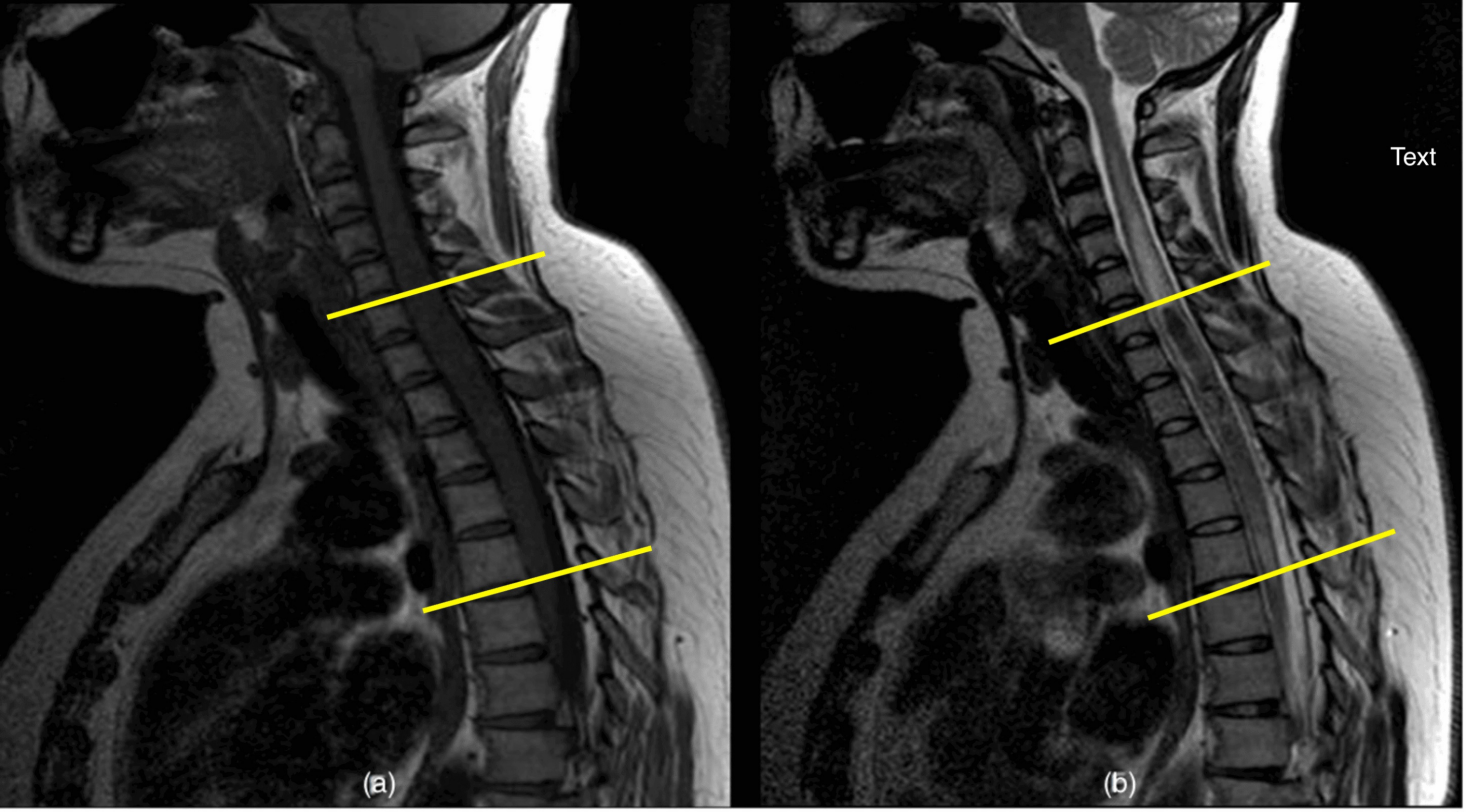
T1 and T2 sequenced MRI images (a) T1 sequenced and (b) T2 sequenced MRI of the sagittal section of the whole spine showing a lesion extending from level C6 down to T4 with associated oedema (described by yellow lines) MRI: Magnetic resonance imaging
